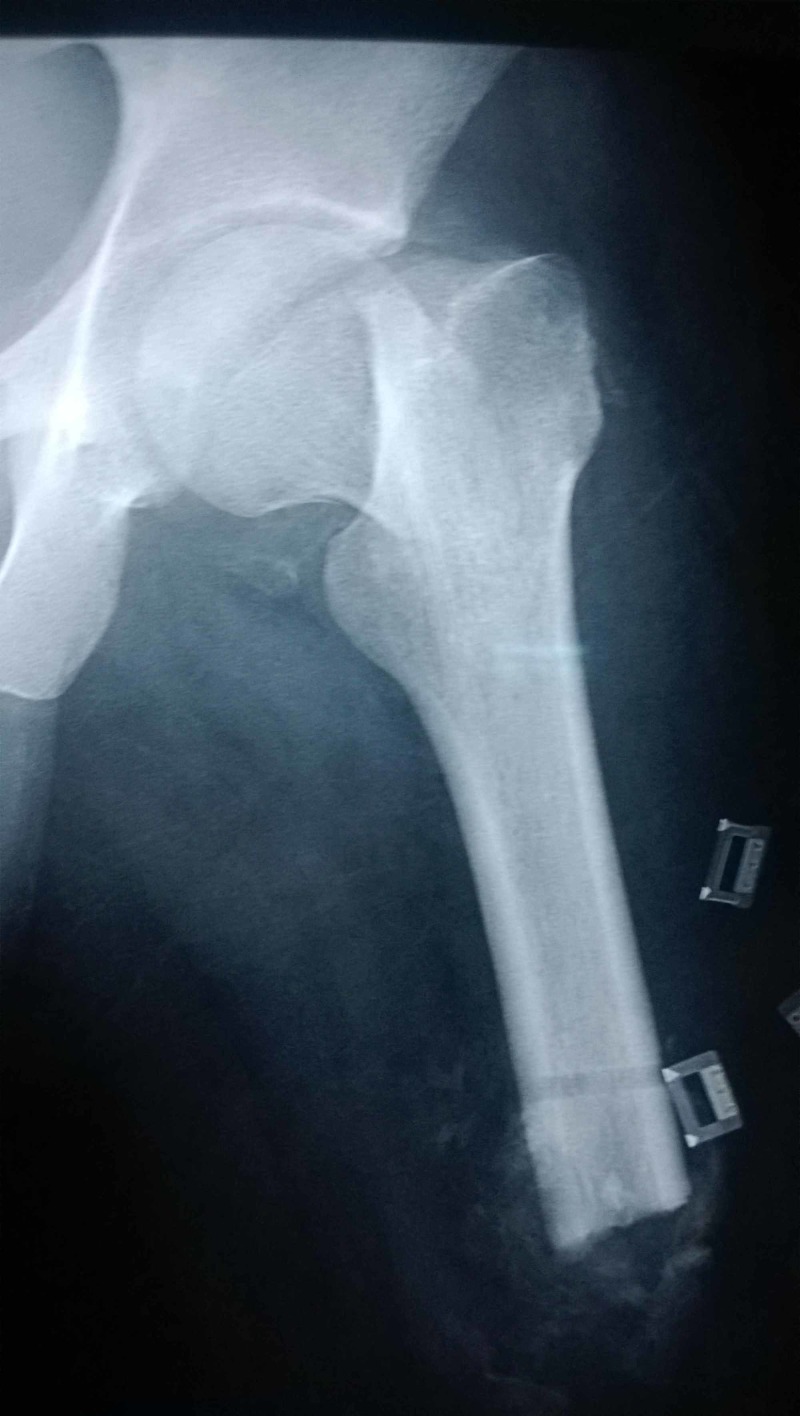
Antero posterior radiograph of patient B with a left sided femoral neck fracture. Note the overriding of the shaft fragment relative to the neck.
